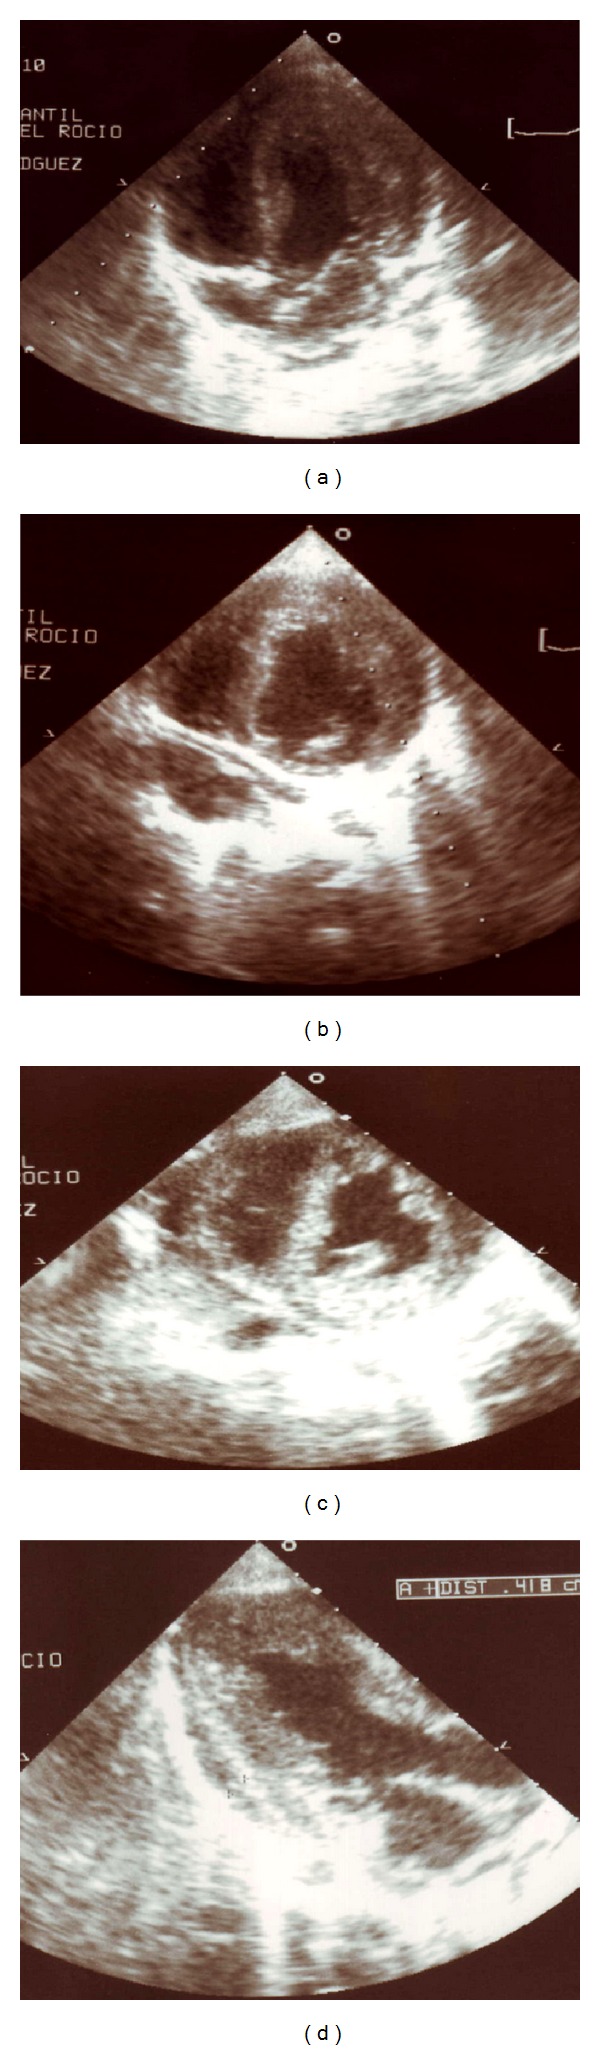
Neonatal echocardiography showing cardiac morphology similar to that in fetal echocardiography.
